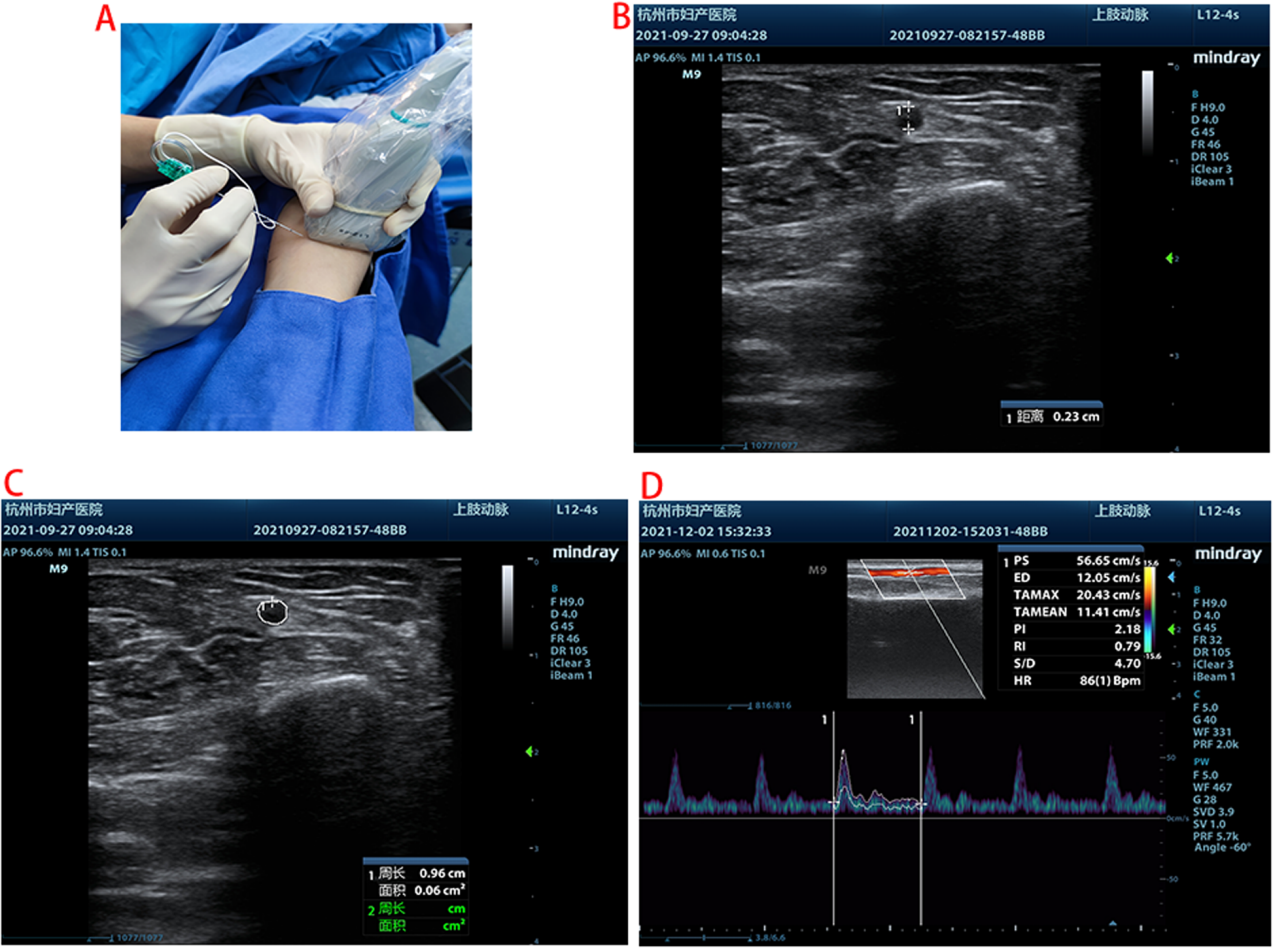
Median nerve block and ultrasonographic measurements
